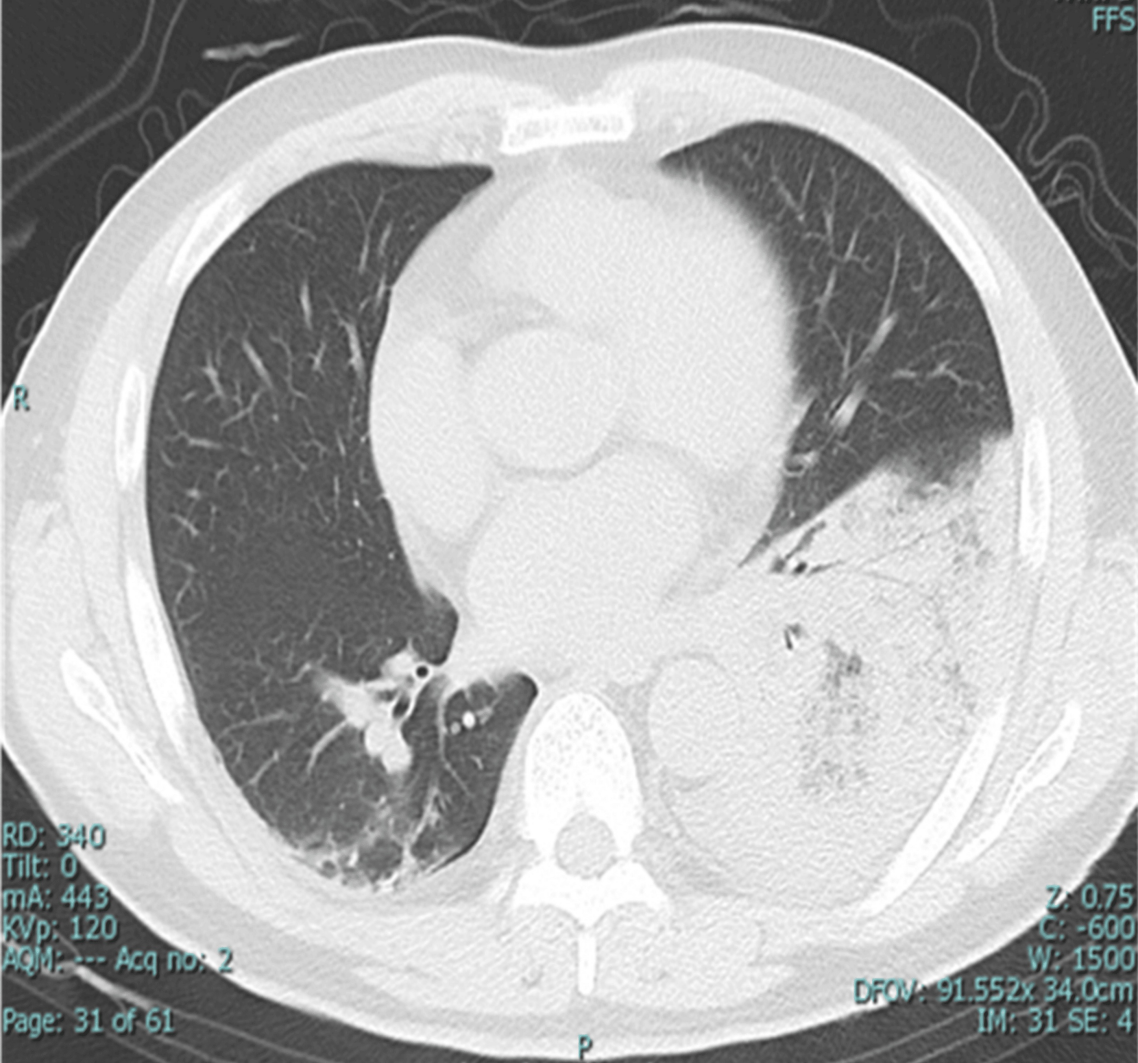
CT of chest revealing diffuse bilateral consolidation opacities in the lower lobes.
